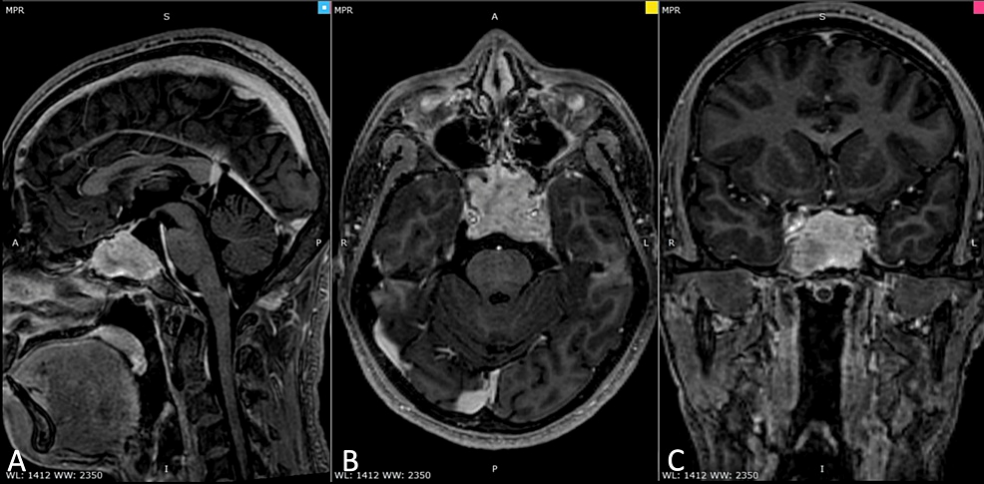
Pre-operative brain MRI T1 with contrast showing an avidly enhancing, lobulated, irregularly shaped sellar mass measuring 3.8 x 3.3 x 2.3 cm (AP x W x CC) extending superiorly and inferiorly, eroding the dorsum of the sella and the upper clivus. (A) Sagittal view, (B) Axial view, and (C) Coronal view.
